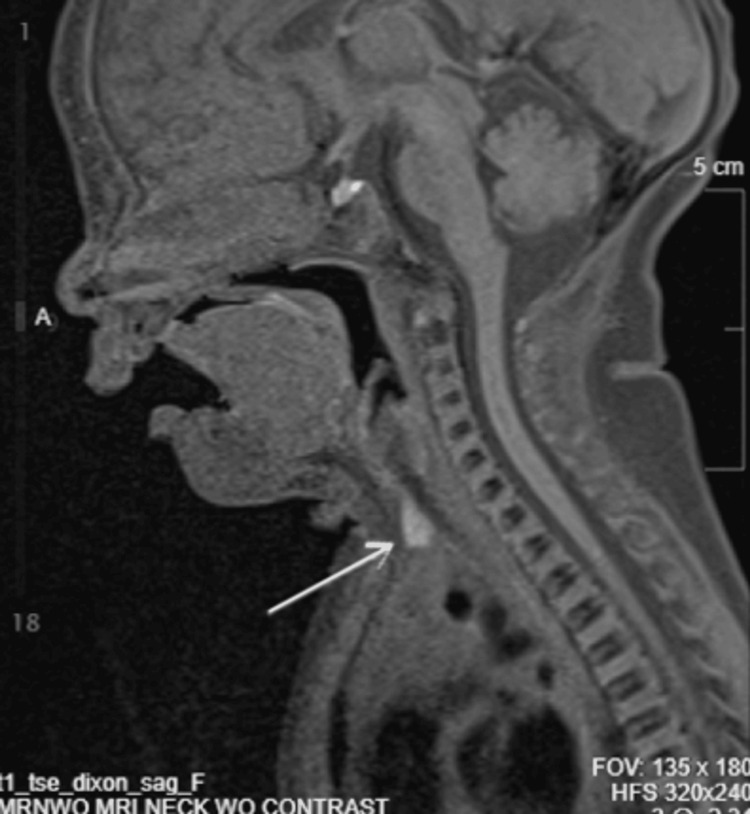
Sagittal MRI of the neck demonstrating a superficial infrahyoid midline lesion (arrow) without evidence of deep extension, consistent with congenital midline cervical cleft. MRI: magnetic resonance imaging
